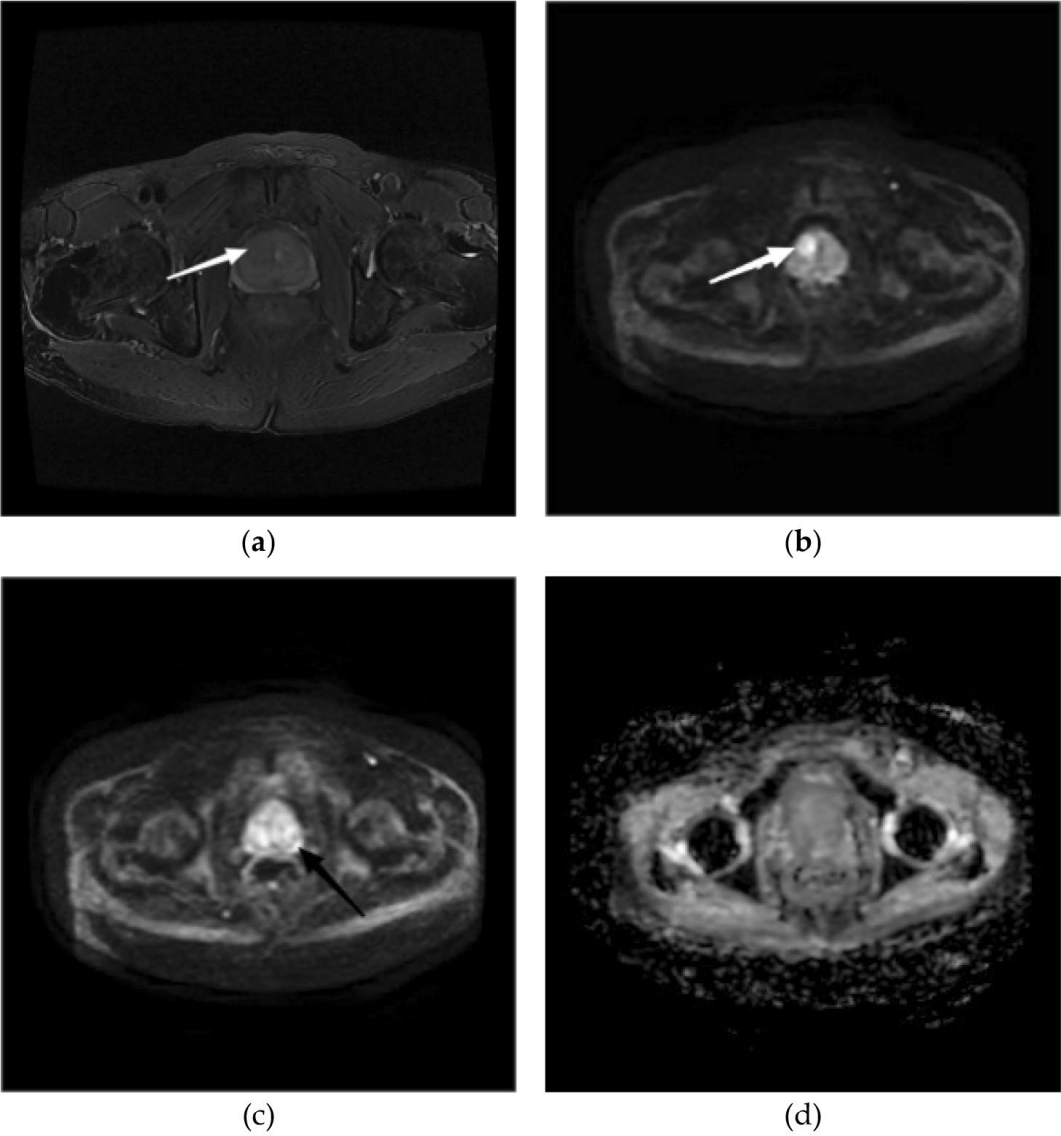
An 80-year-old patient with a PI-RADS v2.1 score of 3 + 3. Transition zone (TZ): (a) Axial T2WI shows a heterogeneous signal intensity with obscured margins nodule (white arrow). (b) DWI map shows a focal lesion with a markedly hyperintense signal (white arrow) corresponding to the lesion seen in (a). T2WI PI-RADS = 3, DWI PI-RADS = 4, PI-RADS assessment category = 4. Peripheral zone (PZ): (c) DWI map shows a focal lesion with a markedly hyperintense signal (black arrow) in the right PZ. (d) Apparent diffusion coefficient (ADC) map does not show a markedly hypointense signal corresponding to the lesion seen in (c). DWI PI-RADS = 3, ADC PI-RADS = 1, PI-RADS assessment category = 3.
